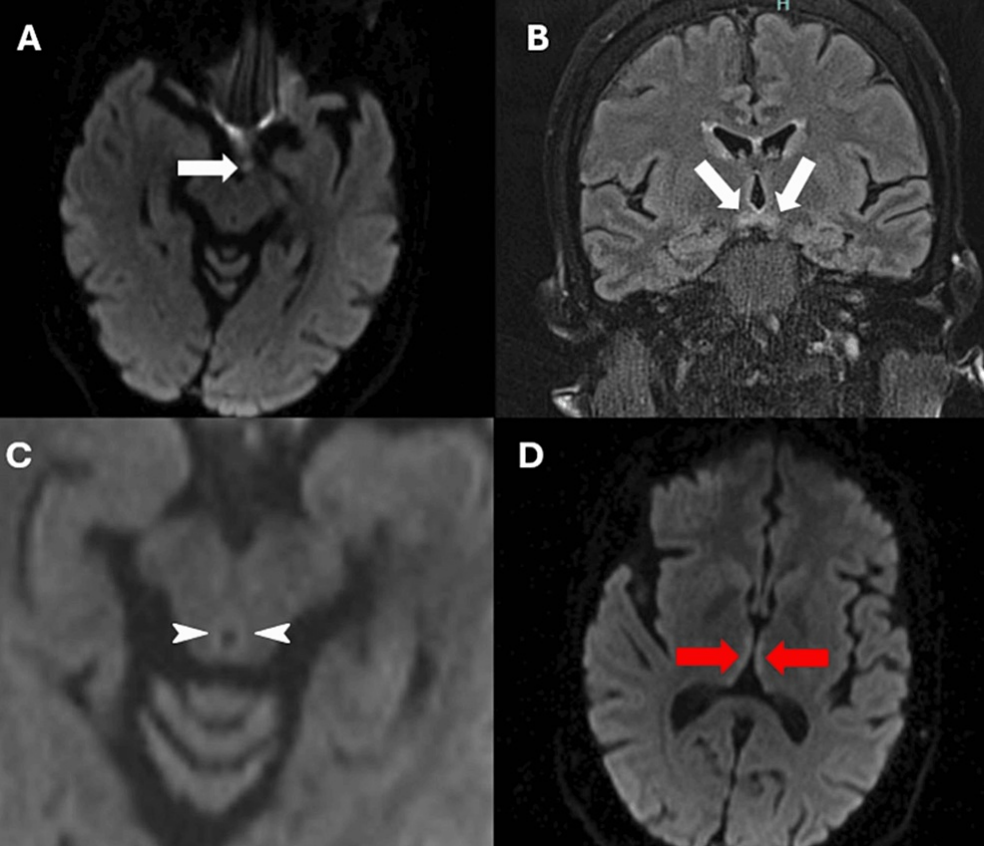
Brain MRI with and without contrast. A: Axial diffusion-weighted imaging (DWI) sequence showing diffusion restriction in mamillary bodies (white arrows). B: Coronal flair sequence showing hyperintensity in mamillary bodies (white arrows). C: Axial DWI sequence showing diffusion restriction in the periaqueductal gray matter (white arrows head). D: DWI showing diffusion restriction in the medial thalamic nuclei (red arrows).
